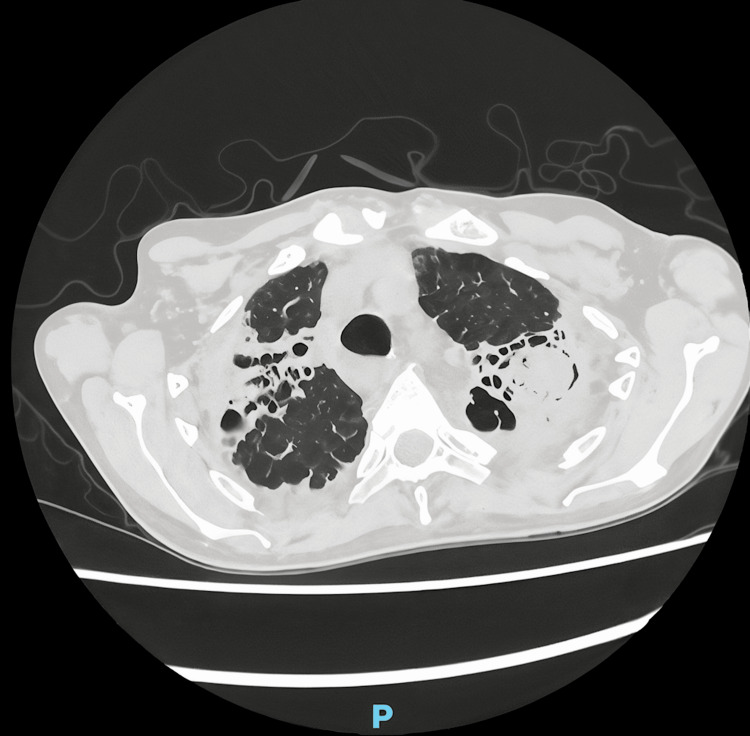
High-resolution CT scan The image shows a left-sided aspergilloma and right-sided worsening varicose bronchiectasis.
